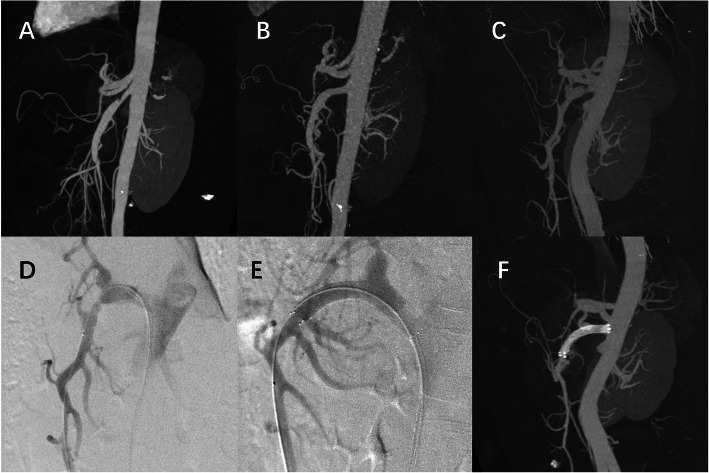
Two cases of SISMAD patients. a & b A patient of Yun’s type IIa who had incomplete remodeling after 1 year of conservative treatment (a before treatment; b 1 year after discharge). c, d, e, and f A patient of Yun’s type IIa who had successful endovascular treatment and showed good stent patency during follow-up (c & d CTA and digital subtraction angiography [DSA] before the intervention; e DSA after stent implantation; f follow-up CTA after 2 years)
